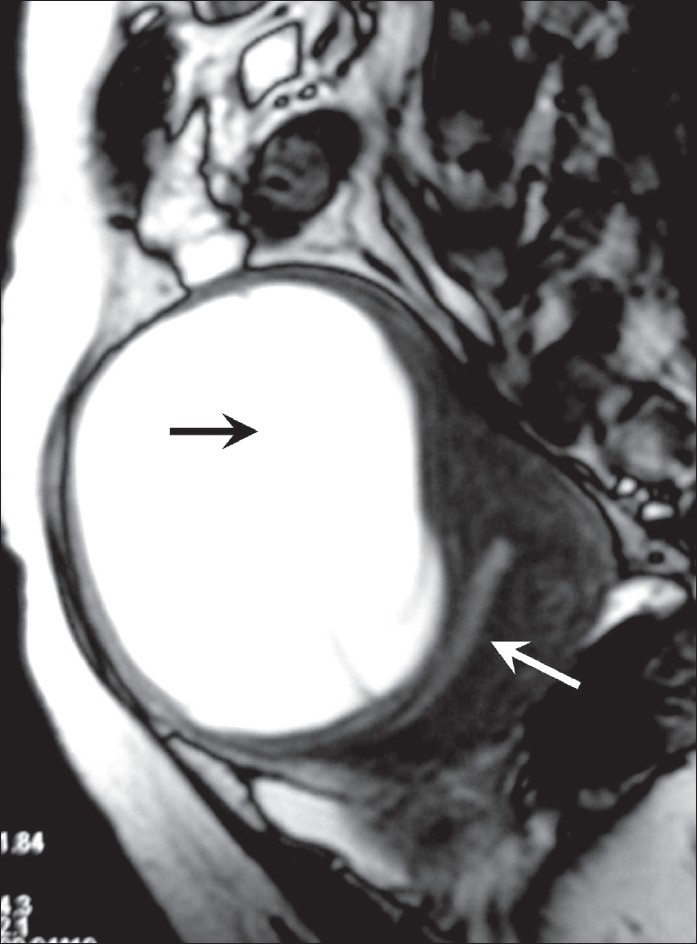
Gradient-echo MRI in the sagittal section shows a posteriorly displaced endometrial stripe (white arrow) and a cystic mass in the anterior myometrium (black arrow)
